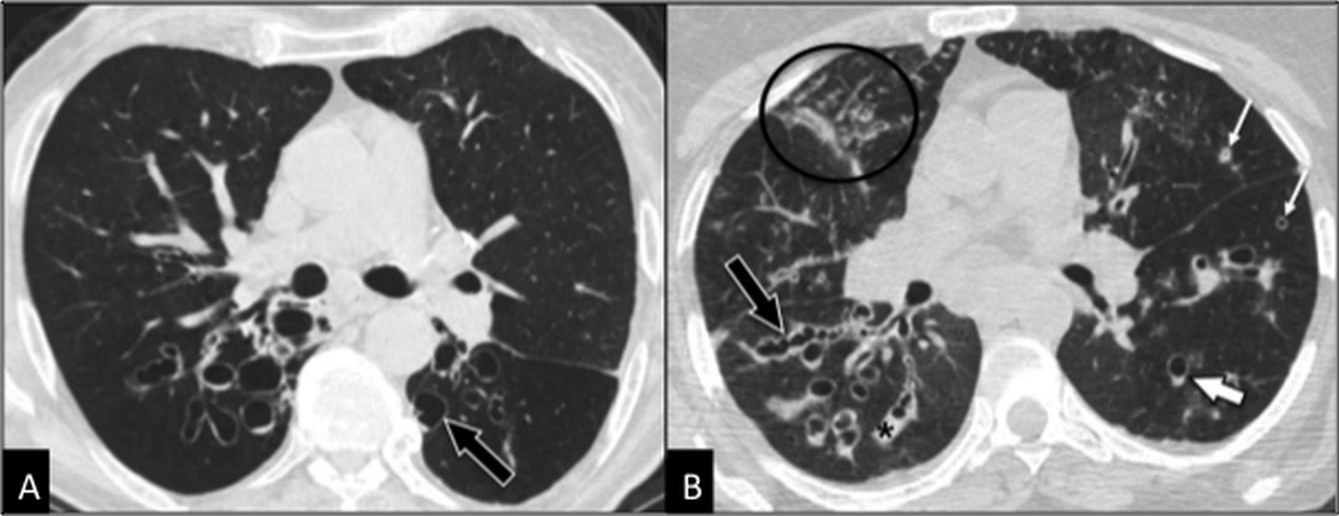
Clinically relevant airway disease Comparison between bronchiectases (A) and bronchiolitis due to underlying bronchiectases (b). CT is diagnostic in both cases showing in the first case (A) dilated bronchi with cystic appearance *(arrow)* whereas in the second case (B) dilated bronchi with cylindrical and varicose *(black arrow)* appearance, together with bronchial mucoid impaction *(black asterisk)*, thickening of bronchial walls *(thin white arrows)*, centrilobular nodules and consolidations *(circle)*. The patchy and asymmetric distribution of the above-mentioned findings is typical of infectious bronchiolitis. Note the “signet ring sign” *(thick white arrow)* typical of bronchiectasis, due to the greater diameter of the bronchus in comparison to the adjacent artery.
